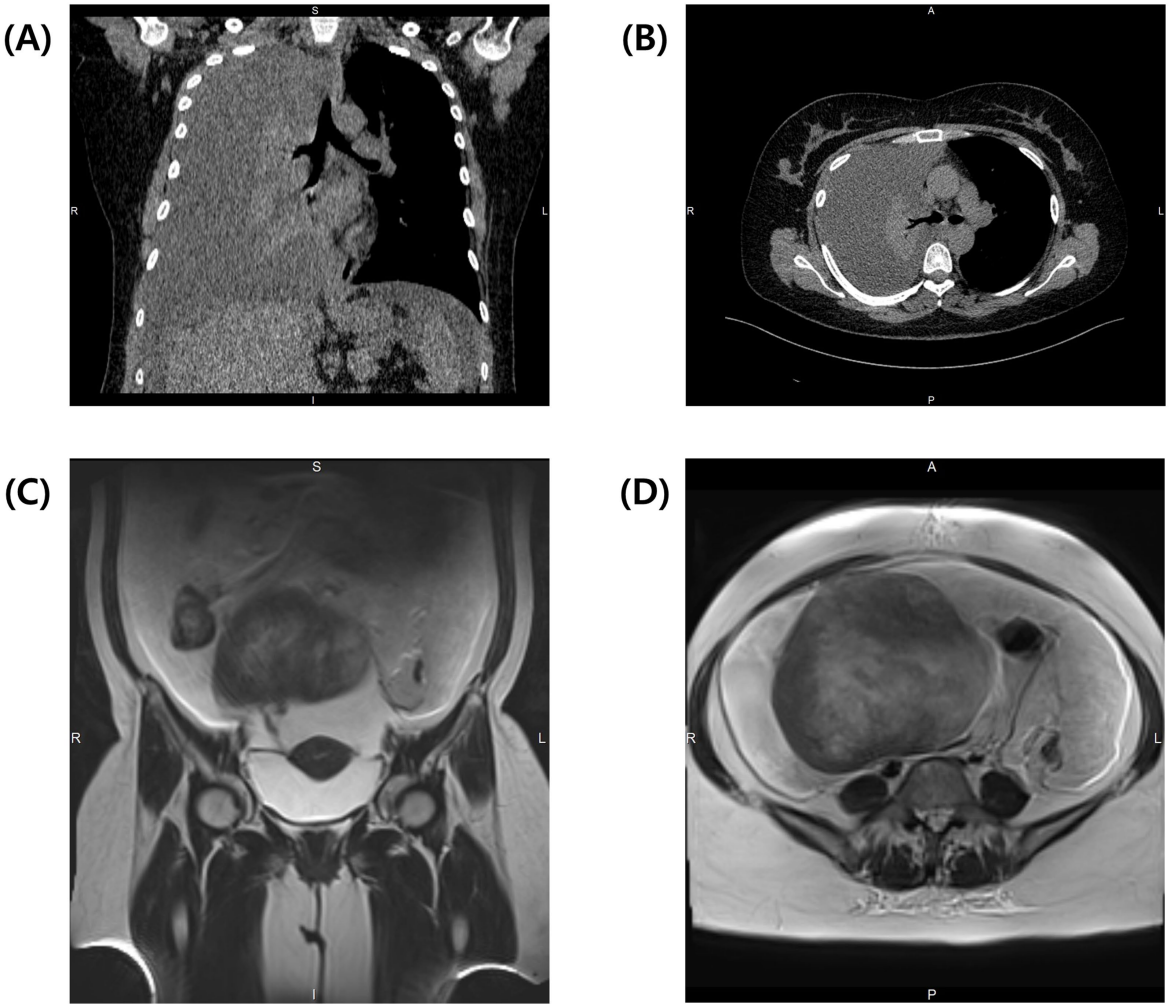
Preoperative CT of the thorax demonstrates complete collapse of the right lung due to massive pleural effusion. (A) Coronal view and (B) transverse view of the thorax at the level of tracheal bifurcation. Preoperative T2-weighted MRI of the abdomen reveals massive ascites and a solid tumor. (C) Coronal view shows the connection between the tumor and the uterus. (D) Transverse view of the abdomen shows a large solid tumor measuring 14.5 × 15.5 × 14.3 cm.
